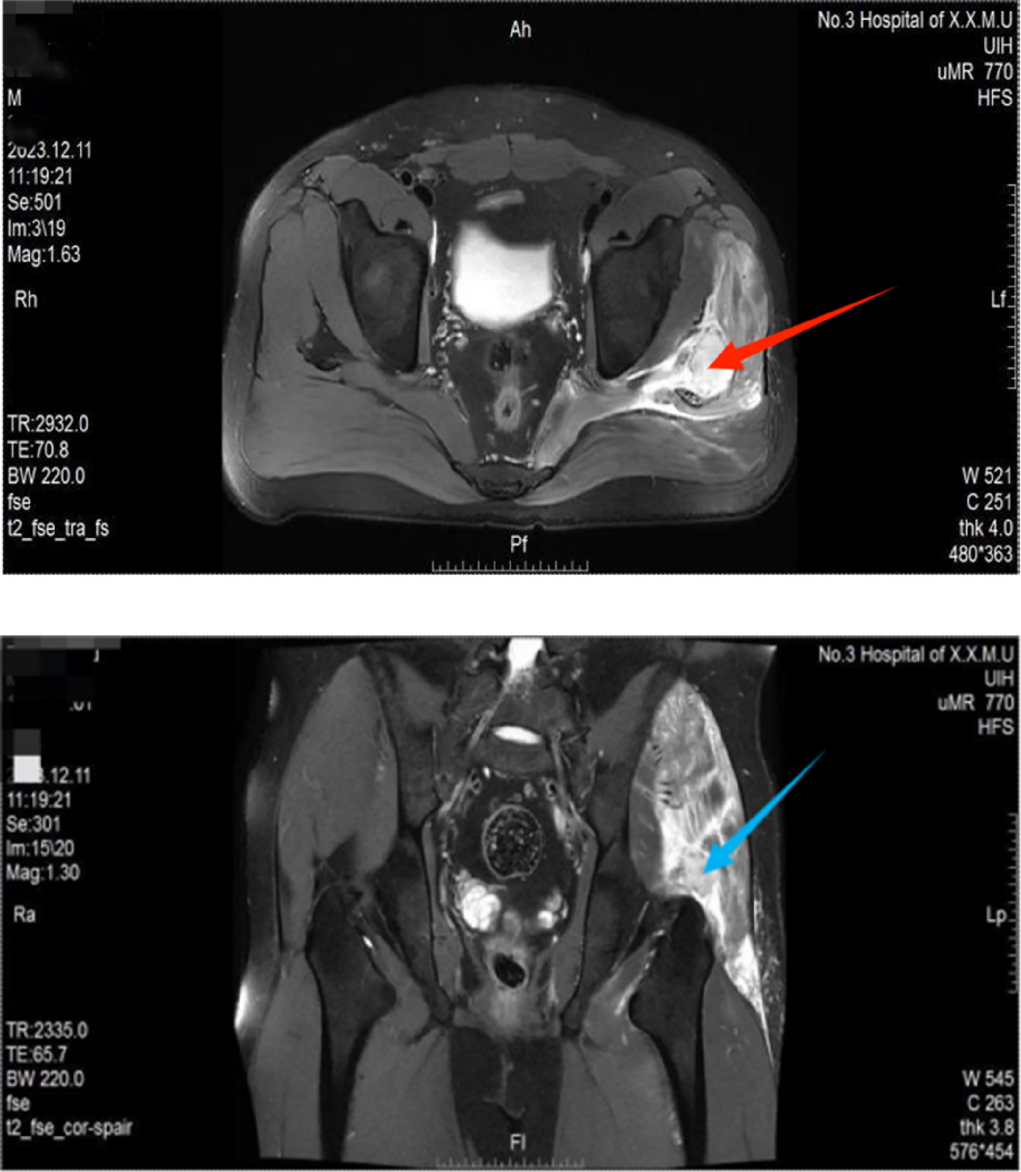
Upper Pic: Abnormal high signal shadow of the left gluteus medius muscle seen in MRI t2_fse_tra_fs (red arrow). Lower Pic: An abnormal enhancement confined to the gluteus medius muscle in MRI t2_fse_cor-spair (blue arrow).
